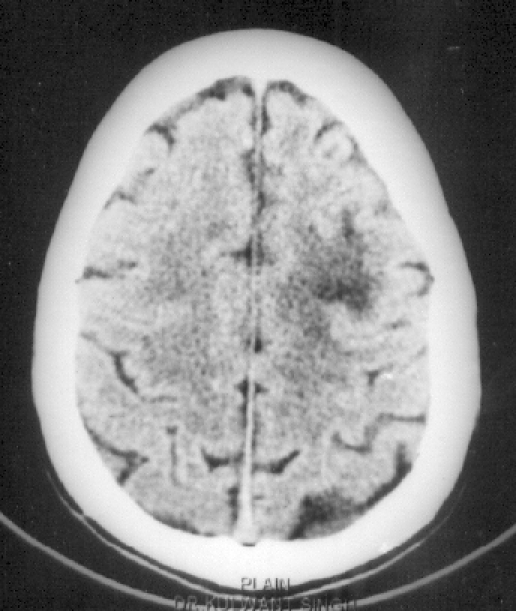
CT scan brain showing a non-enhancing hypodense lesion in the left frontal cortex with sulci prominent in relation to the age.
